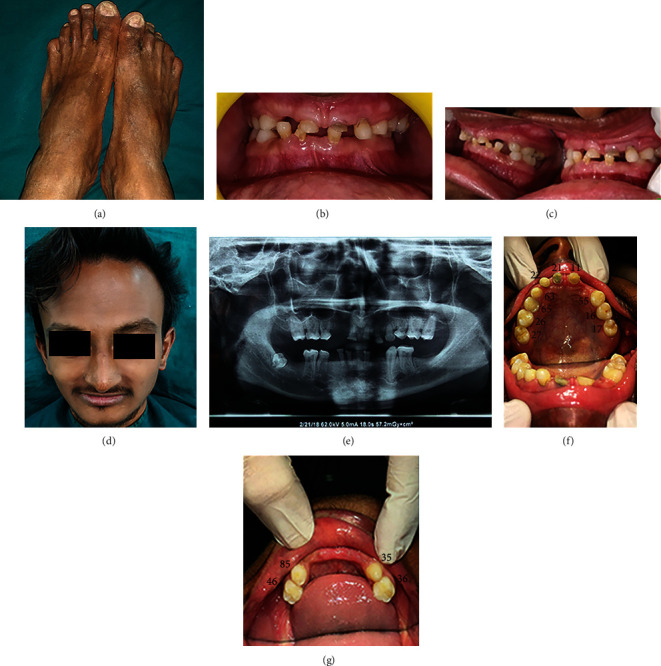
(a) Dystrophy of toe nails, (b–d) pretreatment occlusal vertical dimension loss, (e) pretreatment orthopantomogram, (f) pretreatment maxillary, and (g) mandibular arch.
